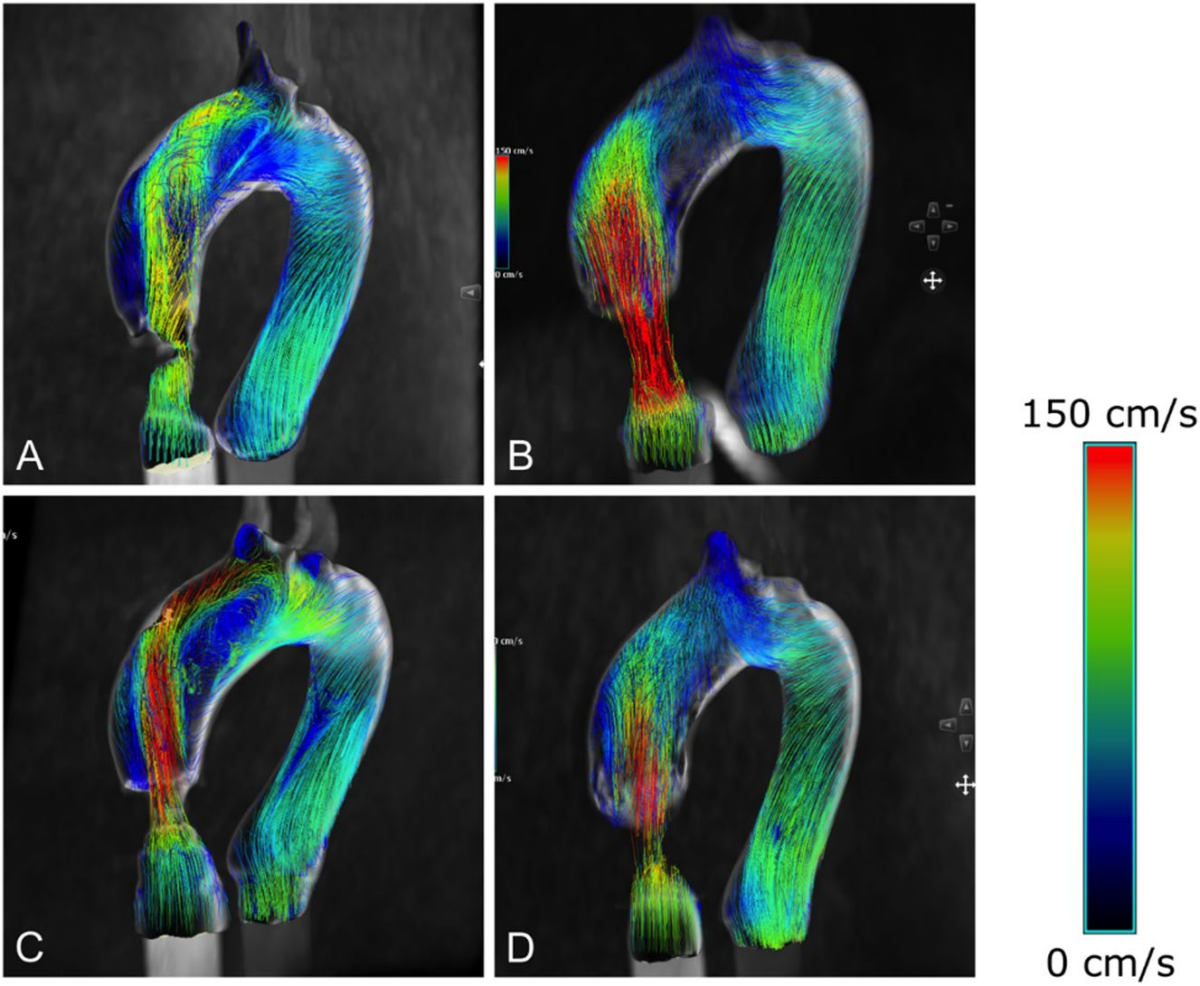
Blood flow patterns within the thoracic aorta visualized with streamlines in anterolateral view using 4D flow Magnetic Resonance Imaging: (A) INTUITY Elite 23 mm; (B) Perceval S; (C) SAPIEN 3 Ultra 23 mm; (D) Evolut PRO + 26 mm.
